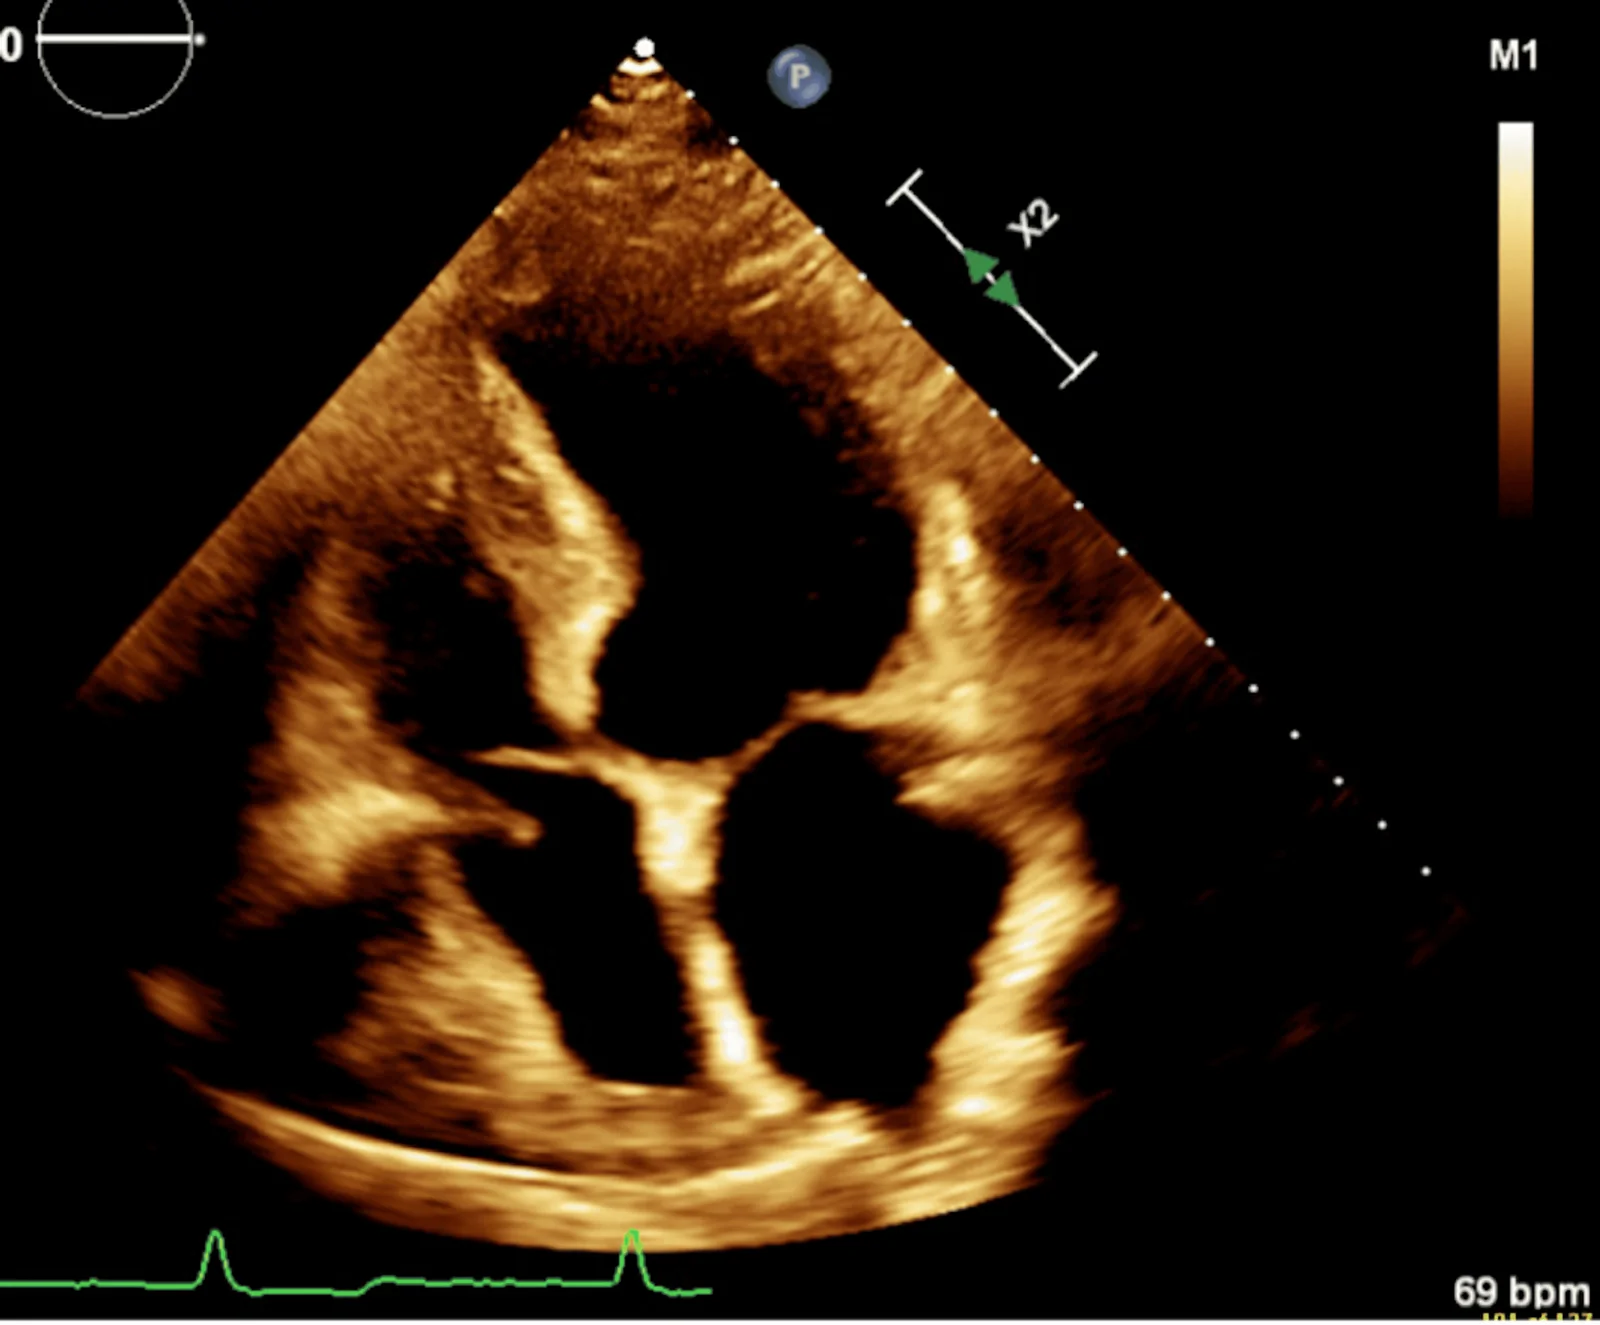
TTE in October 2025 (without ultrasound contrast agent) (A4C view) LV apex not well-visualized, suspected apical thrombus TTE: transthoracic echocardiography, A4C: apical four-chamber, LV: left ventricle
